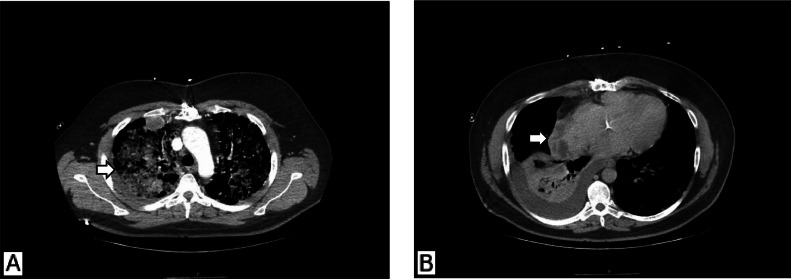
(A) Axial CT chest with contrast showing bilateral dense ground-glass opacities in upper and lower lobes, consistent with diffuse pulmonary hemorrhage (arrow). (B) The right atrial mass shows heterogeneous enhancement and is isoattenuating to the blood pool (arrow).
